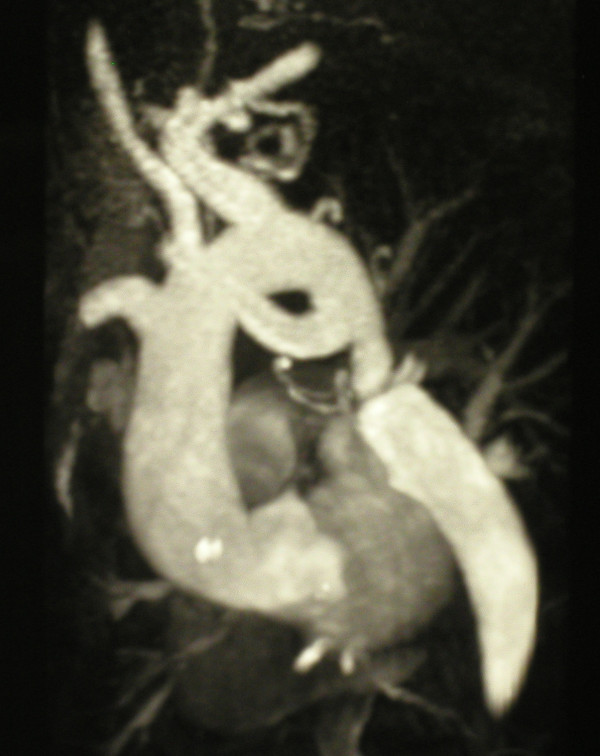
MRI scan showing the re-coarctation.
